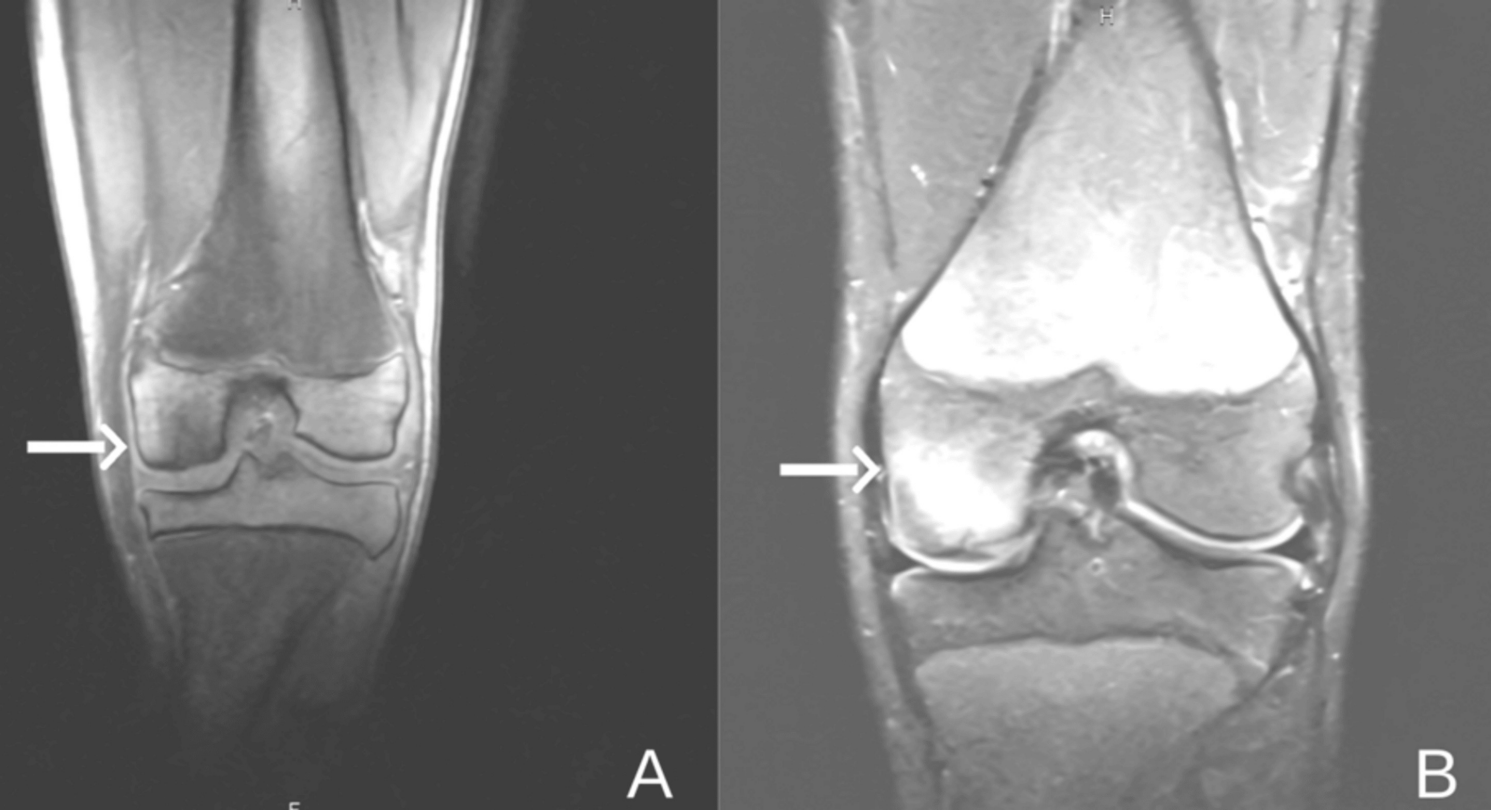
Coronal MRI images of the left knee. (A) T1-weighted image shows an osteochondral defect involving the medial femoral condyle (white arrow), characterized by focal disruption of the subchondral bone. (B) The corresponding T2-weighted image highlights bone marrow edema in the same region (white arrow), consistent with an active lesion. MRI, magnetic resonance imaging
